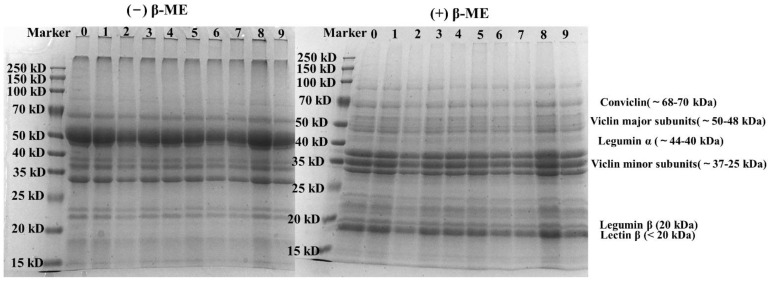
SDS-PAGE of CPI samples modified with different ultrasonic treatment conditions; 0-Unsonicated CPI; 1–3: 10 min sonication group (from 200 W to 600 W); 4–6: 15 min sonication group (from 200 W to 600 W); 7–9: 30 min sonication group (from 200 W to 600 W).
